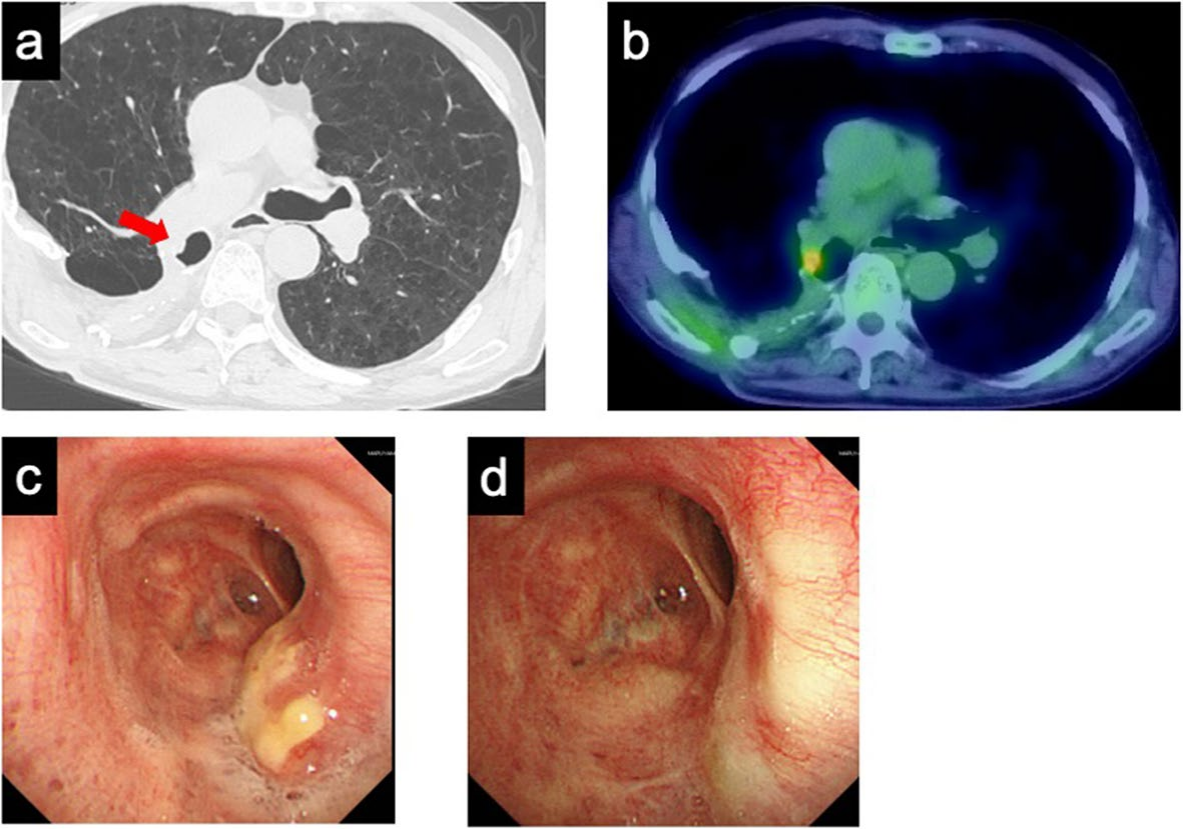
a CT findings showed the endobronchial metastasis at the truncus intermedius (arrow). b PET-CT revealed an uptake in the lesion [SUV_max_ 3.8]. Bronchoscopic image of c before irradiation and d after irradiation
